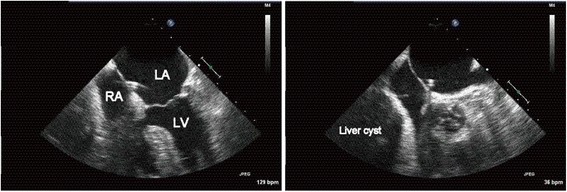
Transesophageal echocardiogram revealed that the giant liver cyst compressed the right atrium and the pacemaker lead was abnormally running to the left atrium through the patent foramen ovale. *LA* left atrium, *LV* left ventricle, *RA* right atrium
